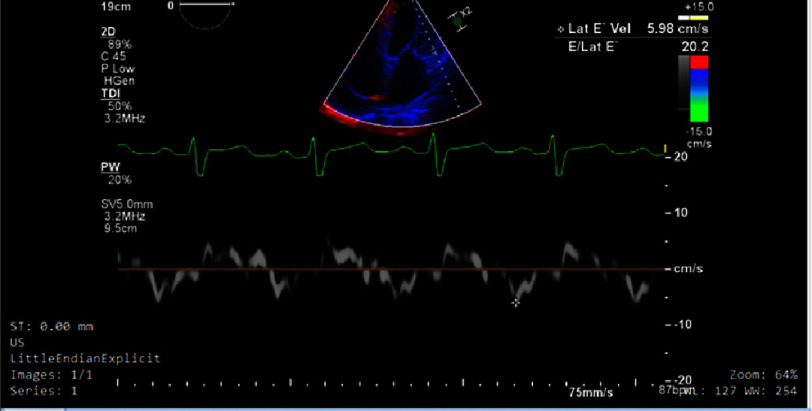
Tissue Doppler imaging (TDI) of the lateral mitral annulus demonstrating an early diastolic velocity (E’) of 5.98 cm/s, indicative of impaired myocardial relaxation.
